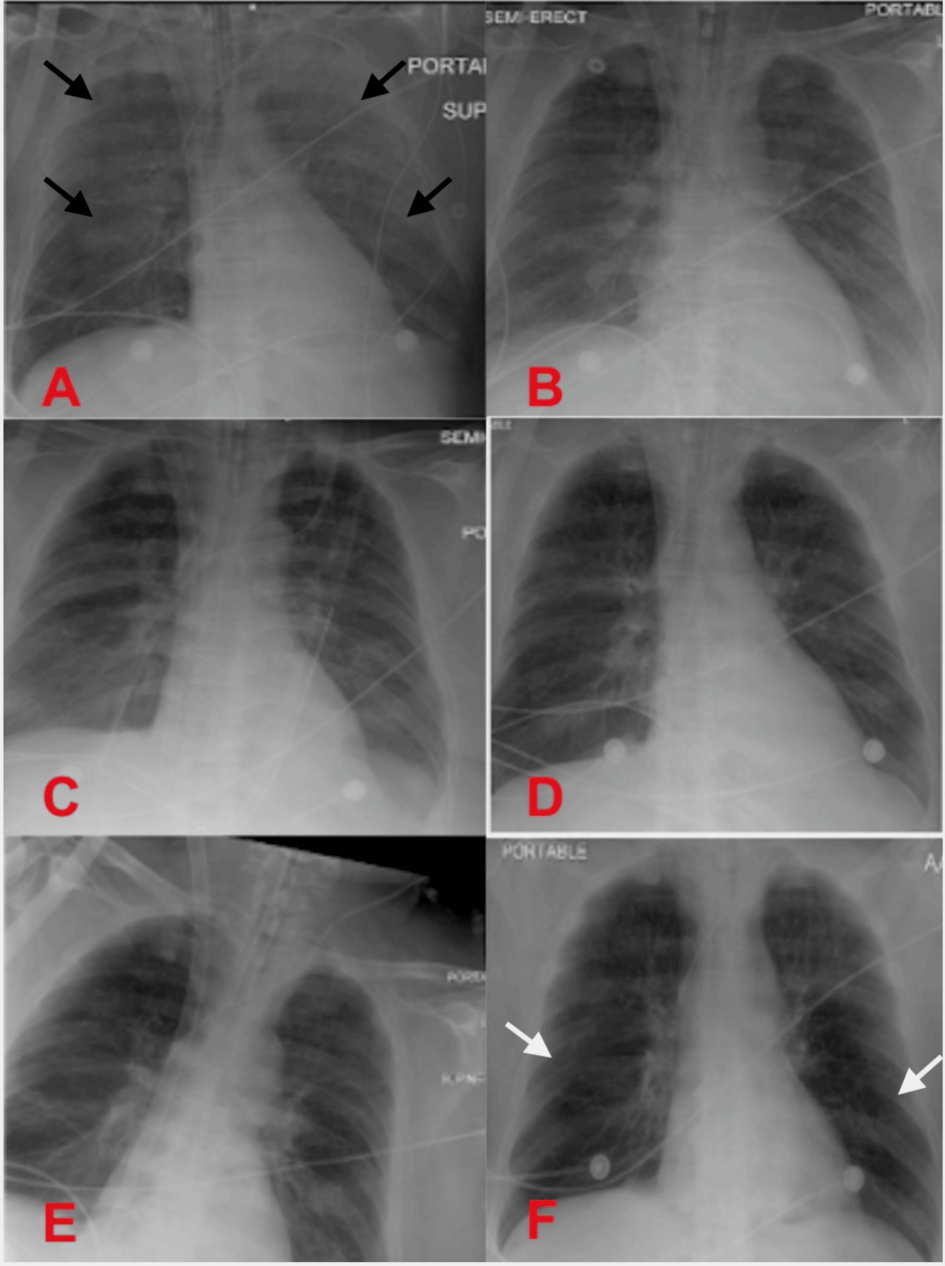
Serial chest X-rays while on ECMO showing interval gradual improvement of initial bilateral infiltrates (black arrows) till complete resolution (white arrows) on Day-7 (A) Day-0, (B) Day-2, (C) Day-3, (D) Day-4, (E) Day-6, and (F) Day-7 at time of decannulation ECMO: extracorporeal membrane oxygenation
